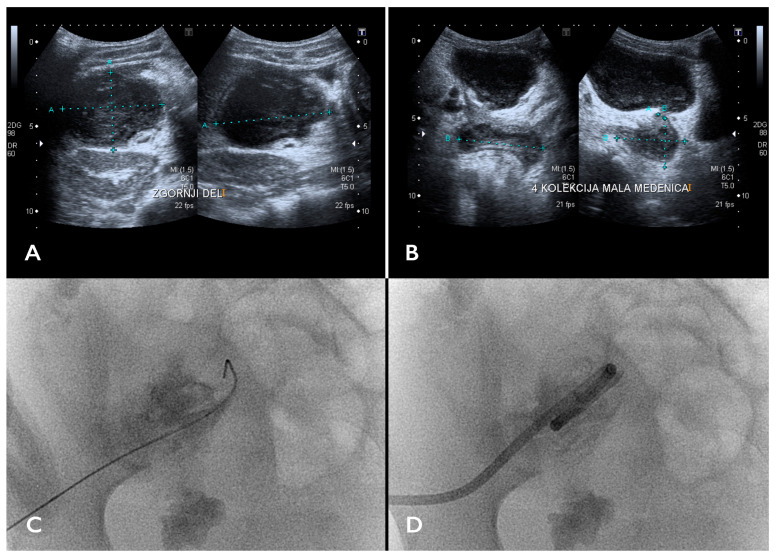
T (A) An ultrasound image showing an encapsulated fluid collection in the right hemiabdomen. (B) The caudal-most part of the multiloculated collection extended retrovesically. (C,D) Fluoroscopic image of image-guided percutaneous abscess drainage (IPAD) using a 10F drainage catheter.
